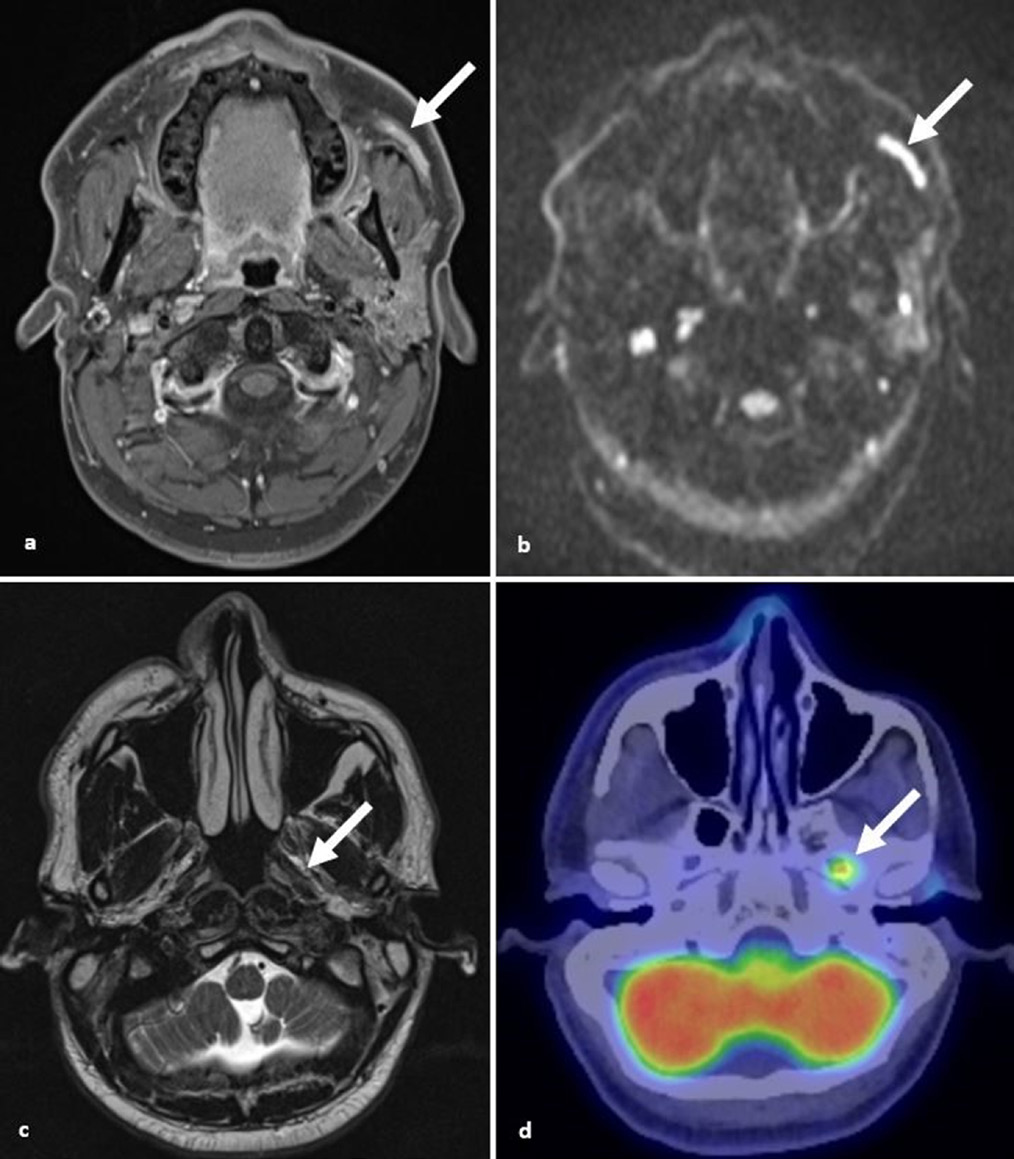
Malignant melanoma. (a, b) Axial T1 post-gadolinium fat saturated and DWI images demonstrate thickening and enhancement of the left buccal branch of the facial nerve with restricted diffusion (arrow) due to previous cutaneous melanoma (arrows). (c) Plaque of intermediate T2 signal extending within the retromandibular region representing spread along the auriculotemporal nerve (arrow). (d) Axial PET CT 6 months later showing uptake at the foramen ovale due to extension along the mandibular nerve (arrow). DWI, diffusion-weighted imaging; PET, positron emission tomography.
